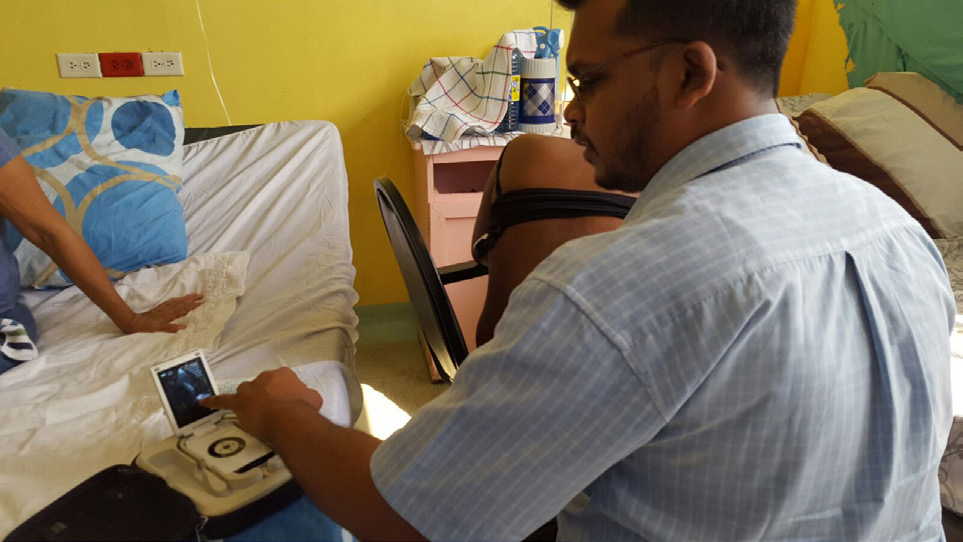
Use of bedside ultrasound by resident.
